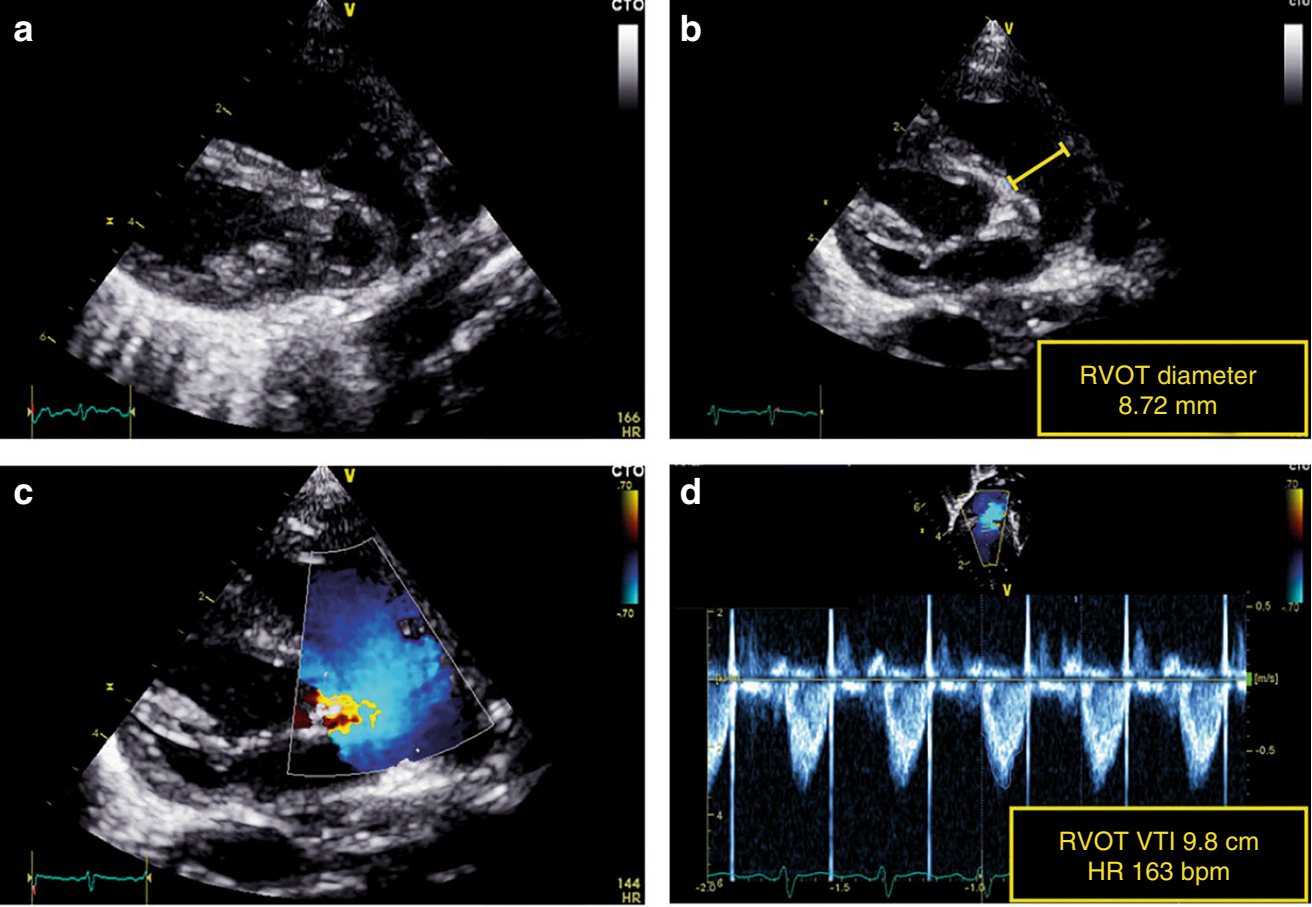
Assessment of right ventricular output (RVO). RV outflow tract diameter is measured from the tilted parasternal long-axis view (a). The diameter measurement should be performed at the pulmonary valve insertion (b). The velocity–time integral (VTI) is acquired in the same view or in the parasternal short-axis view, depending on angle orientation (c). The pulsed wave Doppler recording is paused to obtain a smooth VTI envelope for exact tracing of the signal (d)
